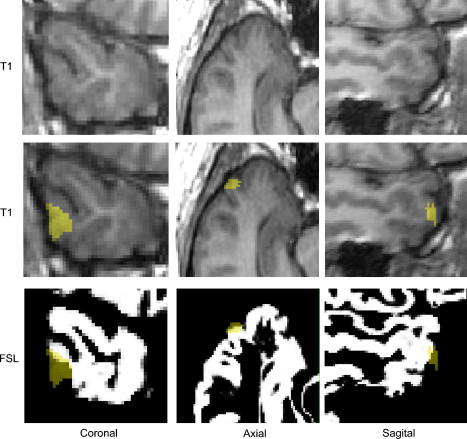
Top panel shows zoomed T1-weighted images and bottom panel shows grey matter class images (created using FSL-FAST) of the right temporal lobe in patient 15. The ROI (transparent yellow to allow visualisation of underlying tissue) is overlaid on the T1-weighted (in the correct location) and the segmented images (part of the ROI appears outside the brain, 57.6%). (For interpretation of the references to colour in this figure legend, the reader is referred to the web version of the article.)
